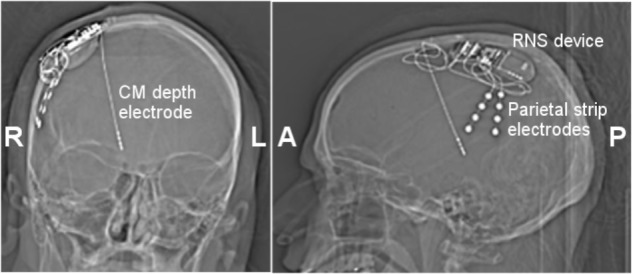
Coronal (Left) and lateral (Right) skull X-ray images. Three 4 contact electrodes were placed. A right centromedian depth electrode (1.5 mm contact size, 1.5 mm inter-contact spacing) and two parietal strip electrodes. One parietal strip electrode was connected to the RNS device, while the second was left unconnected. The RNS device was placed in an appropriately sized right craniectomy. CM, centromedian thalamus.
